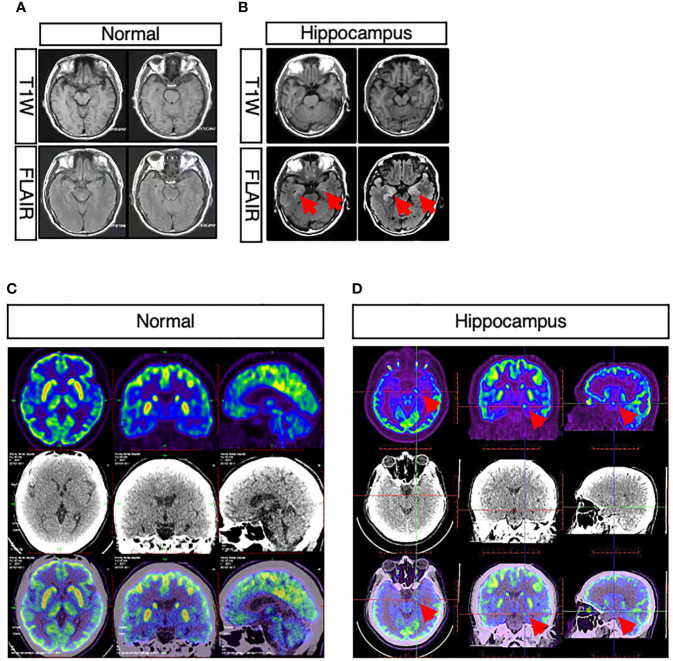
Abnormal neuroimaging findings in patients with CASPR2 antibody-associated encephalitis. (A, B) Comparison between normal and abnormal MRI showed abnormal signals in bilateral hippocampus in patients; (C, D) Comparison between normal and abnormal PET-CT revealed increased metabolism of bilateral basal ganglia and mesial temporal lobe in patients.
